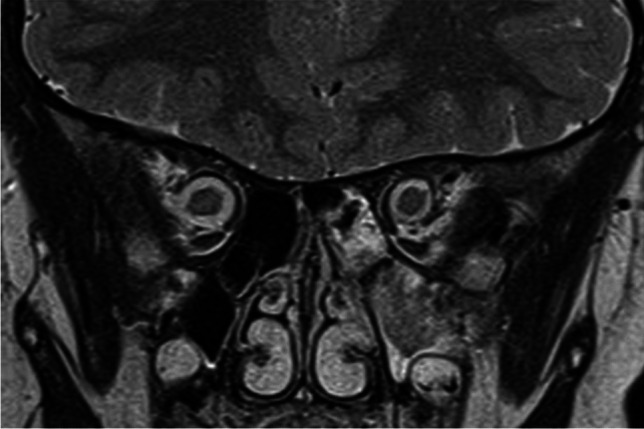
Coronal T2-weighted image shows bilaterally thickened intraorbital optic nerves, consistent with ONGs in a patient with neurofibromatosis type 1 (NF1)
